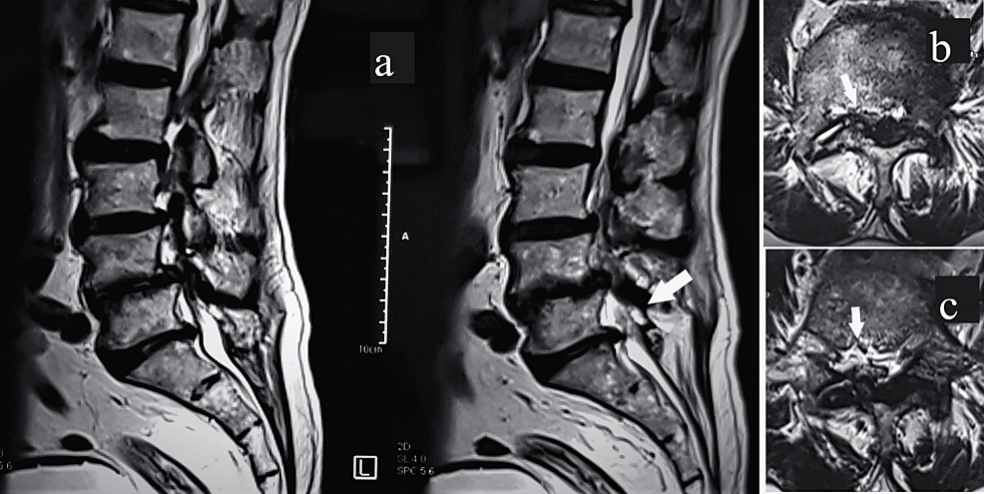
Magnetic resonance imaging of the lumbar spine showing marked spondylo-discoid degenerative changes in sagittal section (a) and canal stenosis at L3-L4 level (b) and L4-L5 disc level (c).
